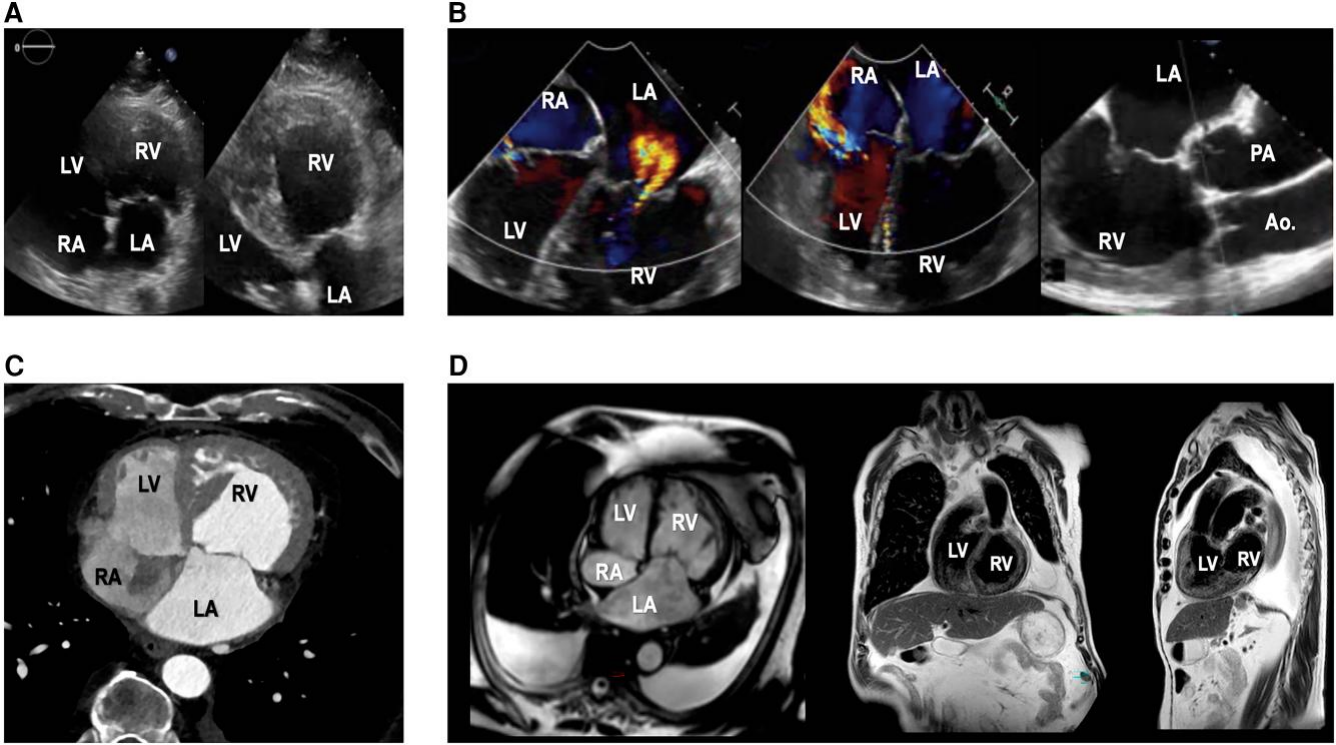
Morphological features in a case of congenitally corrected transposition of the great arteries. Transthoracic (*A*), transoesophageal (*B*), cardiac computed tomography (*C*), and cardiac magnetic resonance imaging (*D*) multimodality imaging in an 84-year-old congenitally corrected transposition of the great arteries patient. Notice the apical displacement of the left-sided tricuspid valve, the parallel course of the great vessels, the morphology of the systemic right ventricle, and the significant regurgitation of both atrioventricular valves. RA, right atrium; RV, right ventricle; LA, left atrium; LV, left ventricle; Ao, aortic artery; PA, pulmonary artery.
